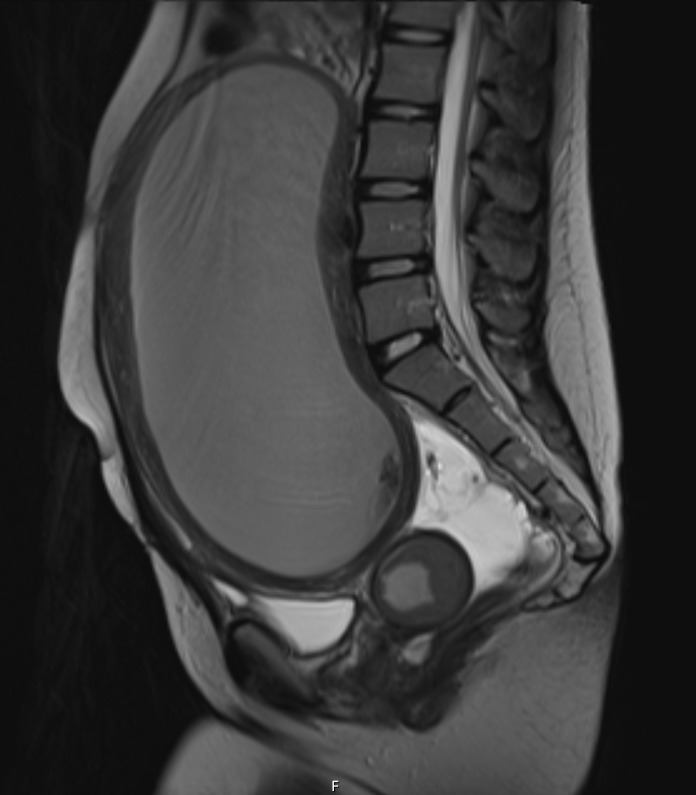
T2 Weighted Sagittal MRI Image.
